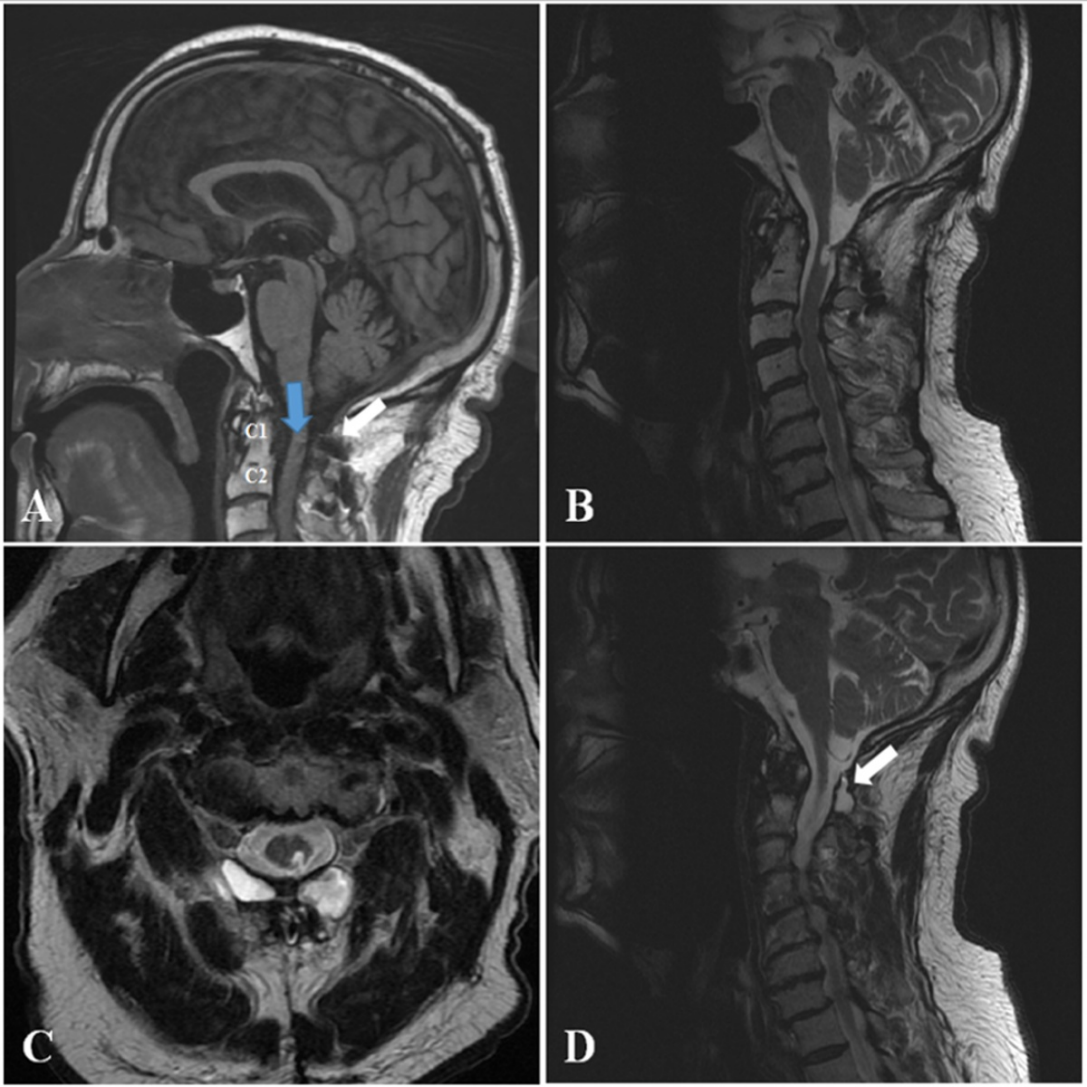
Preoperative Cervical MRI Scan Preoperative magnetic resonance imaging (MRI) scan demonstrating T1 and T2 changes in the cervical spinal canal. (A) T1-weighted non-contrast image of the cervical spinal cord demonstrating a pseudomeningocele (white arrow) and intensity change in the cord between levels C1 and C2 (blue arrow). T2-weighted non-contrast imaging also demonstrates the syrinx accompanying wire encroachment in (B) sagittal and (C) axial views. (D) T2-weighted non-contrast imaging re-demonstrating the pseudomeningocele (arrow) in this patient.
